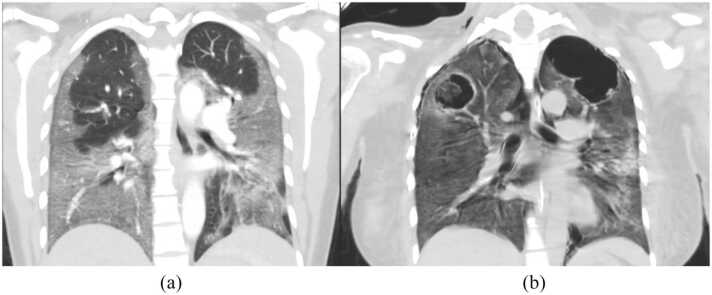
a. CT Chest with contrast on admission. b. CT chest without contrast on hospital day 20.
